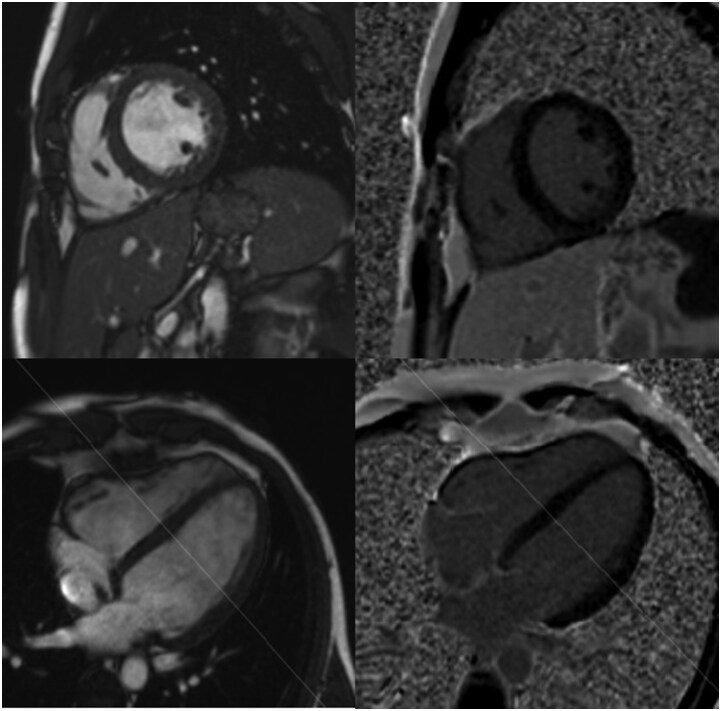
Cardiac magnetic resonance imaging showing absence of late gadolinium enhancement (top right: short axis; bottom right: 4-chamber) and presence of biventricular dilatation (top left: cine short axis; bottom left: cine 4-chamber).
